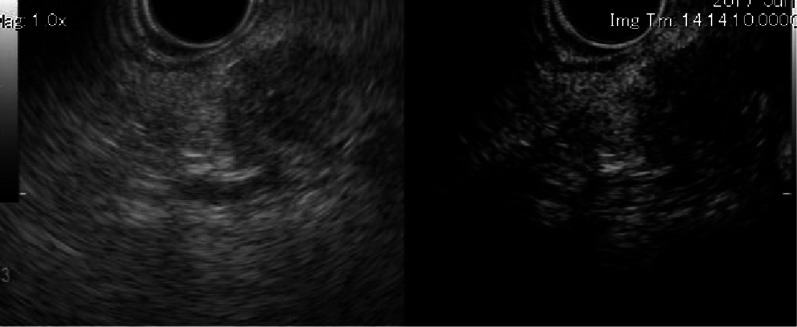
Findings of endoscopic ultrasound (EUS). a EUS reveals a hypoechoic mass measuring 15 × 15 mm in the pancreatic body. b The tumor shows hypoenhancement in the late phase of contrast EUS
